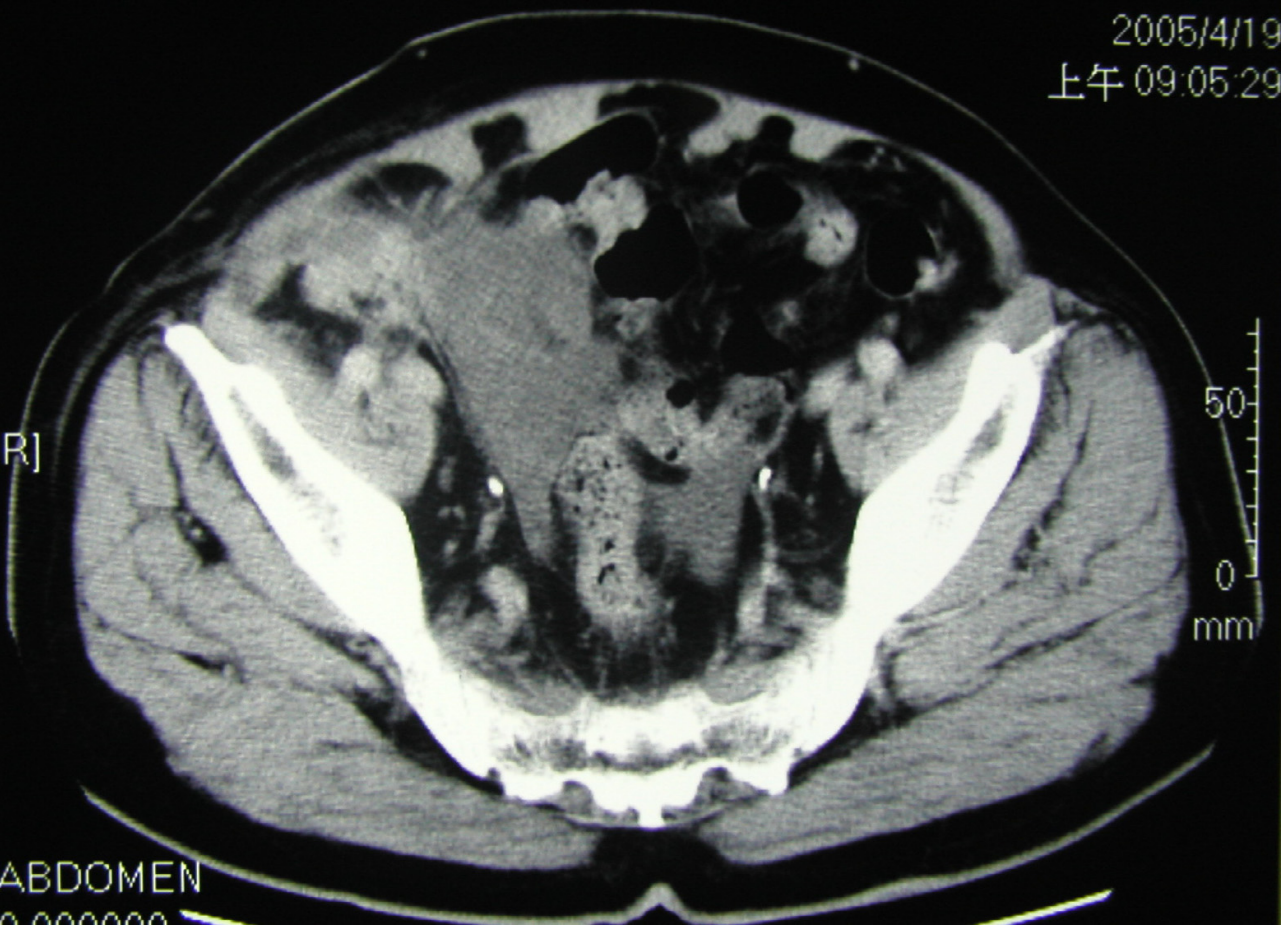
The CT scan showed the irregular hypodense lesion with fat stranding nearby the cecum without obvious enlarged lymph nodes.
